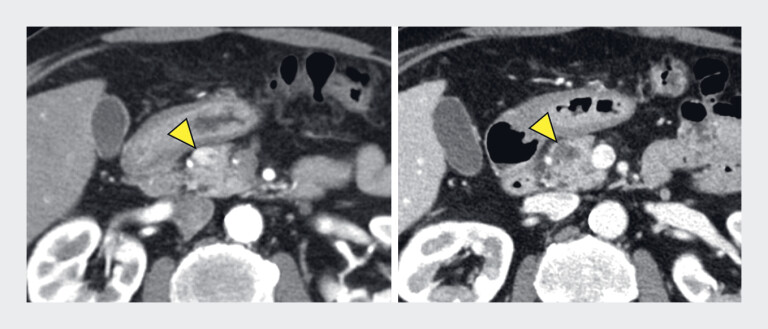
Contrast-enhanced computed tomography (CE-CT) findings before and after EUS-guided ethanol injection (EUS-EI). At the location where the pancreatic tumor was enhanced on CE-CT before EUS-EI (left), no residual tumor was observed after EUS-EI (right), showing that complete ablation was achieved.
